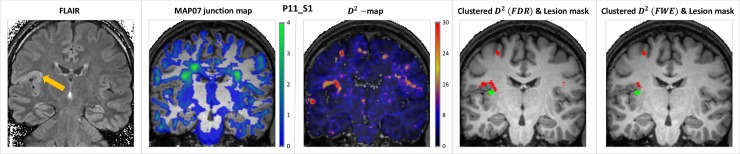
Comparison of the results with FDR and FWE corrected critical values. Thresholded and clustered *D*^*2*^-results overlaid on the T_1_-weighted image of a 27 y.o. male patient with polymicrogyria in the basal region of the left inferior frontal gyrus (see panel D of Fig 8, as well.) Coronal slices presented in neurological orientation, i.e. left side is on the left, slices of the 2D FLAIR image were angulated perpendicular to the hippocampi.
